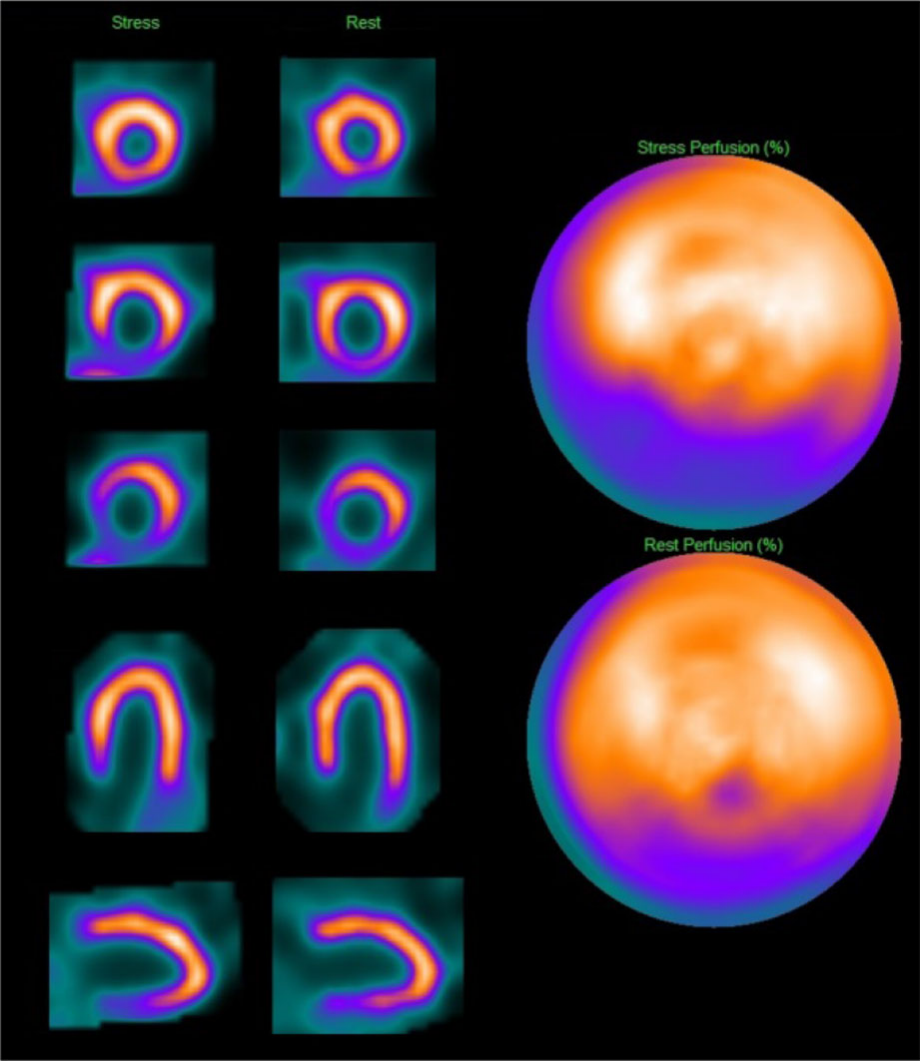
Case 5: Tc-99m tetrofosmin MPS of a 72-year-old man with previous coronary artery bypass graft and severe emphysema showing partial-thickness inferior infarction with mild-to-moderate superimposed and peri-infarct ischaemia. MPS, myocardial perfusion scintigraphy; Tc, technetium
